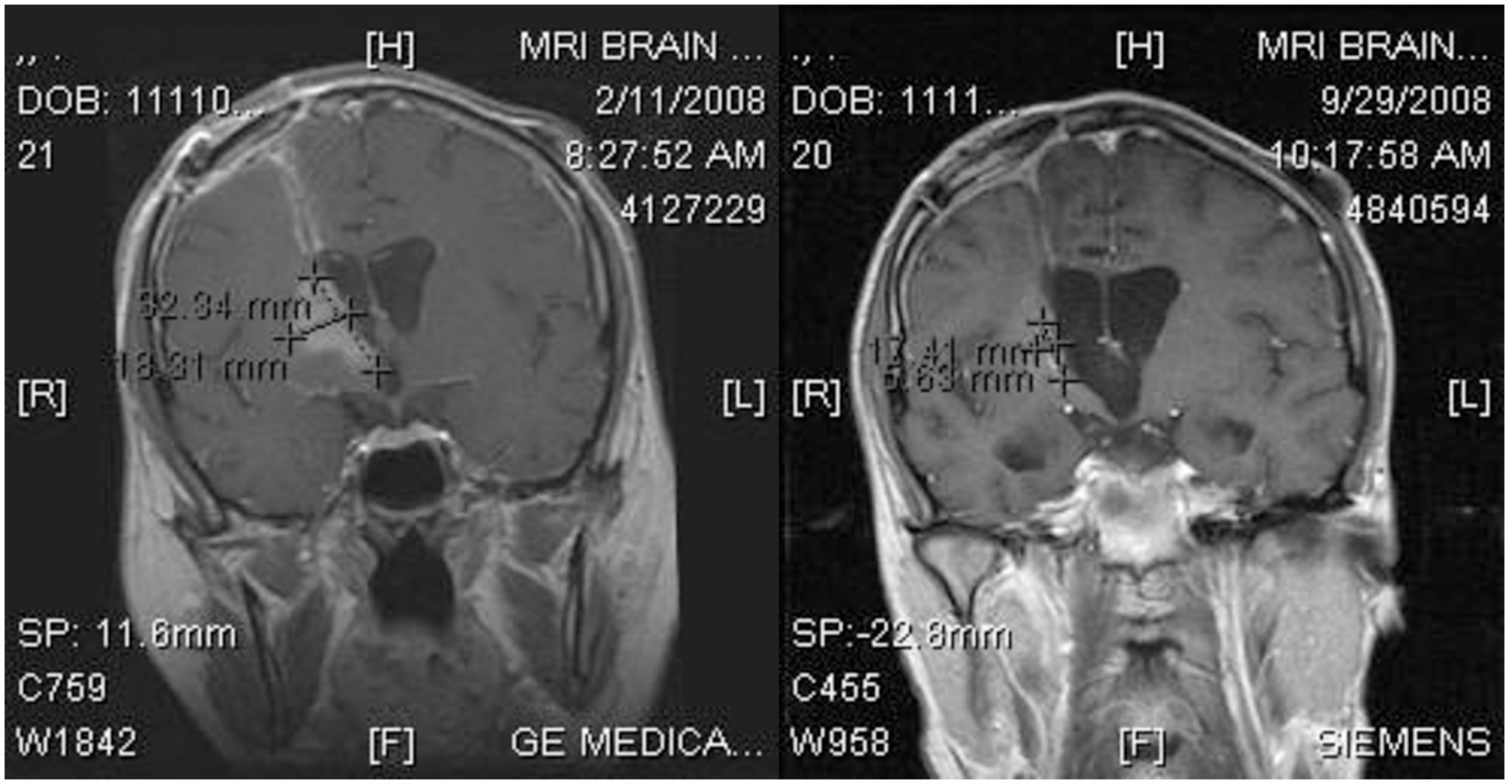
AT-101 single agent objective response in patient with recurrent glioblastoma. The MRI on the left is the post-contrast coronal image before treatment with AT-101. The MRI on the was performed with similar technique after seven months of AT-101 and demonstrates marked reduction in the enhancing abnormality.
